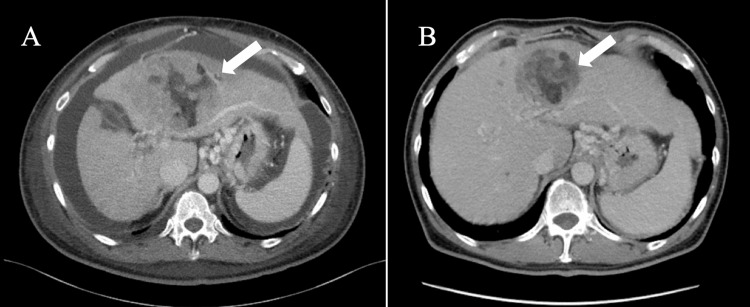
Improved condition after combined immune therapy, as the CT of the transverse plane shows. CT: computed tomography.
